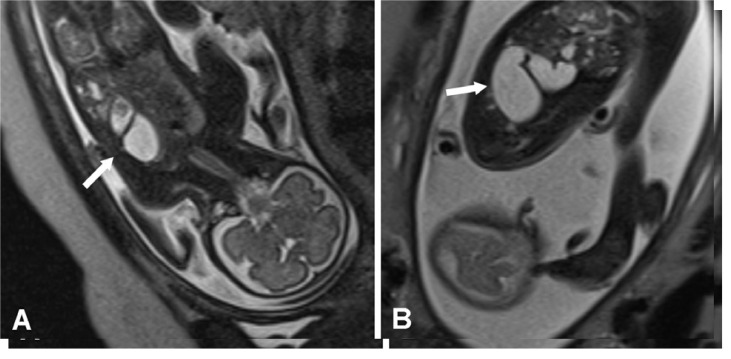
Fet al magnetic resonance imaging showing findings compatible with left-side diaphragmatic hernia (see arrows).
